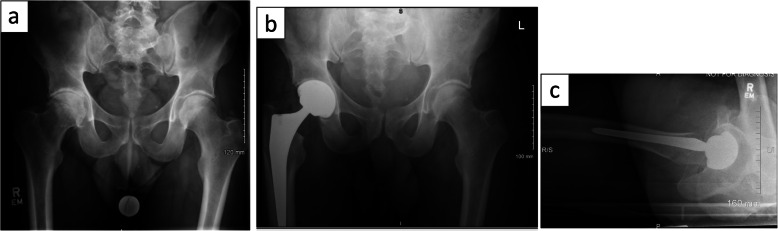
a Pre-operative AP radiograph and b, c post-operative radiographic AP and lateral views of the patient in standing and sitting position showing a satisfactory alignment of the prosthesis
